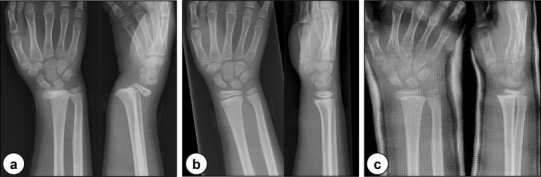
Anteroposterior (left) and lateral (right) radiographs in the initial treatment: a growth plate fracture of the right distal radius; b after closed reduction; and c displacement of the fracture site during cast immobilization
